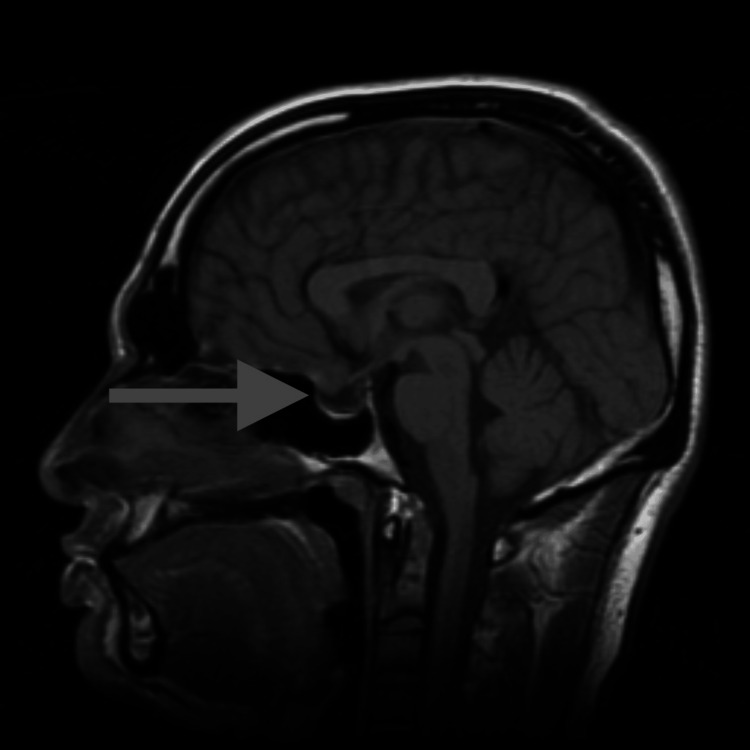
MRI showing non-measurable remains of adenoma after one year of cabergoline treatment.
